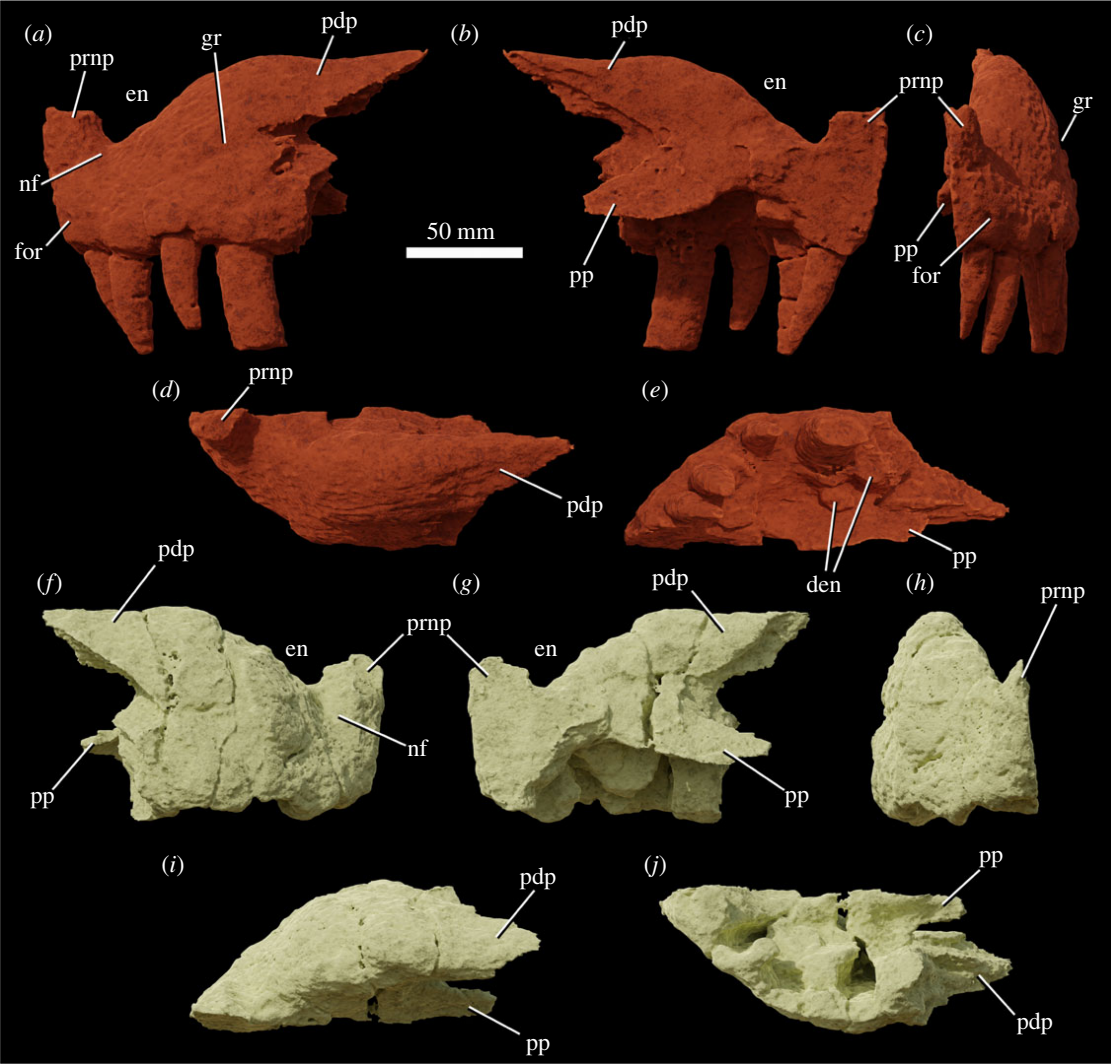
Premaxillae of NHMUK R36620, holotype of *Mambawakale ruhuhu*, reconstructed from CT data. Left premaxilla in lateral (*a*), medial (*b*), anterior (*c*), dorsal (*d*) and ventral (*e*) views. Right premaxilla in lateral (*f*), medial (*g*), anterior (*h*), dorsal (*i*) and ventral (*j*) views. Abbreviations: den, dentary tooth fragments; en, external naris; for, foramen; gr, groove on the lateral surface of the posterodorsal process; nf, narial fossa; pdp, posterodorsal process; pp, palatal process; prnp, prenarial process.
